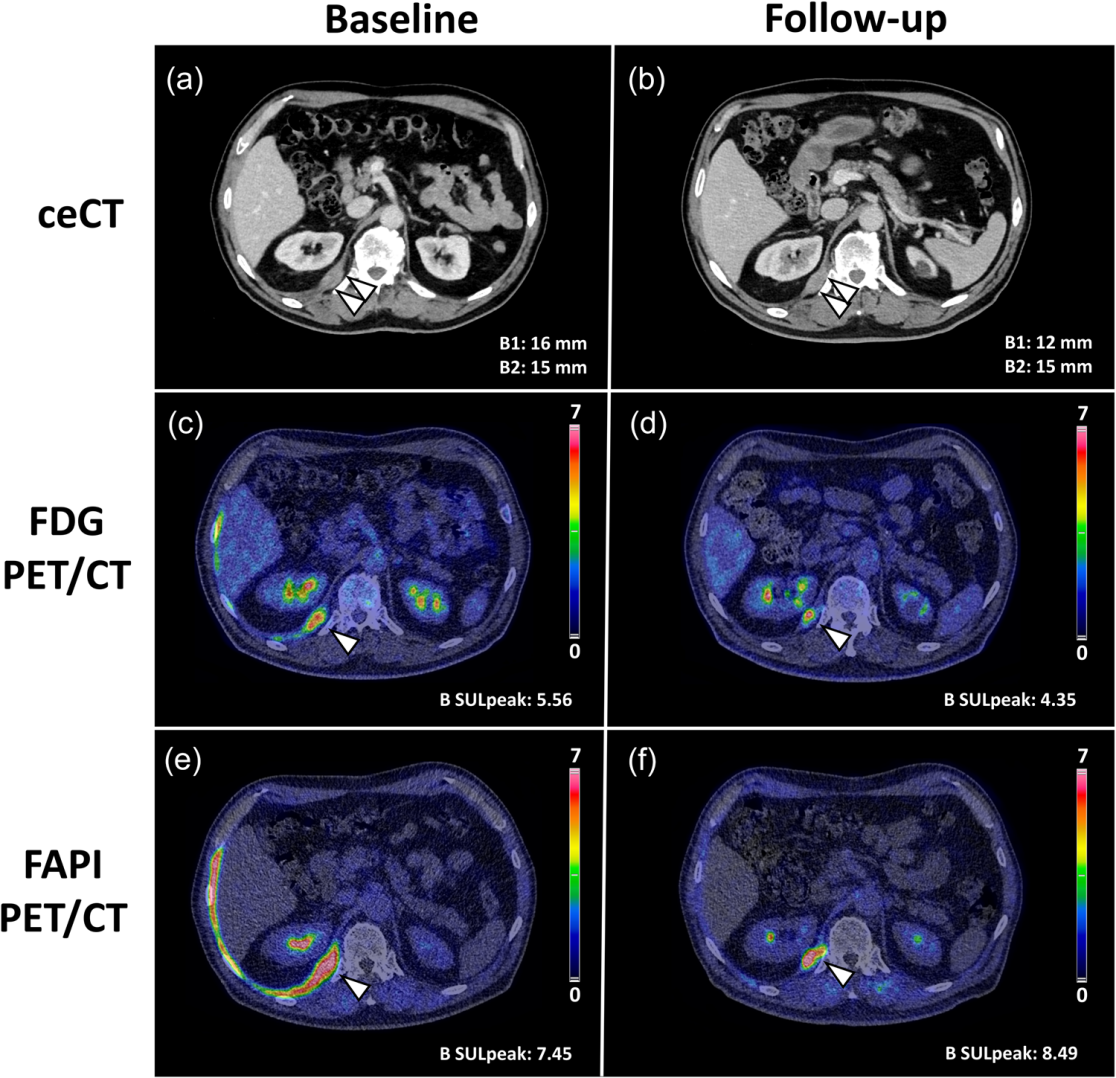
Imaging response assessment of Lesion B, located in the dorsomedial pleura, with ceCT (a, b), FDG PET/CT (c, d), and FAPI PET/CT (e, f).
